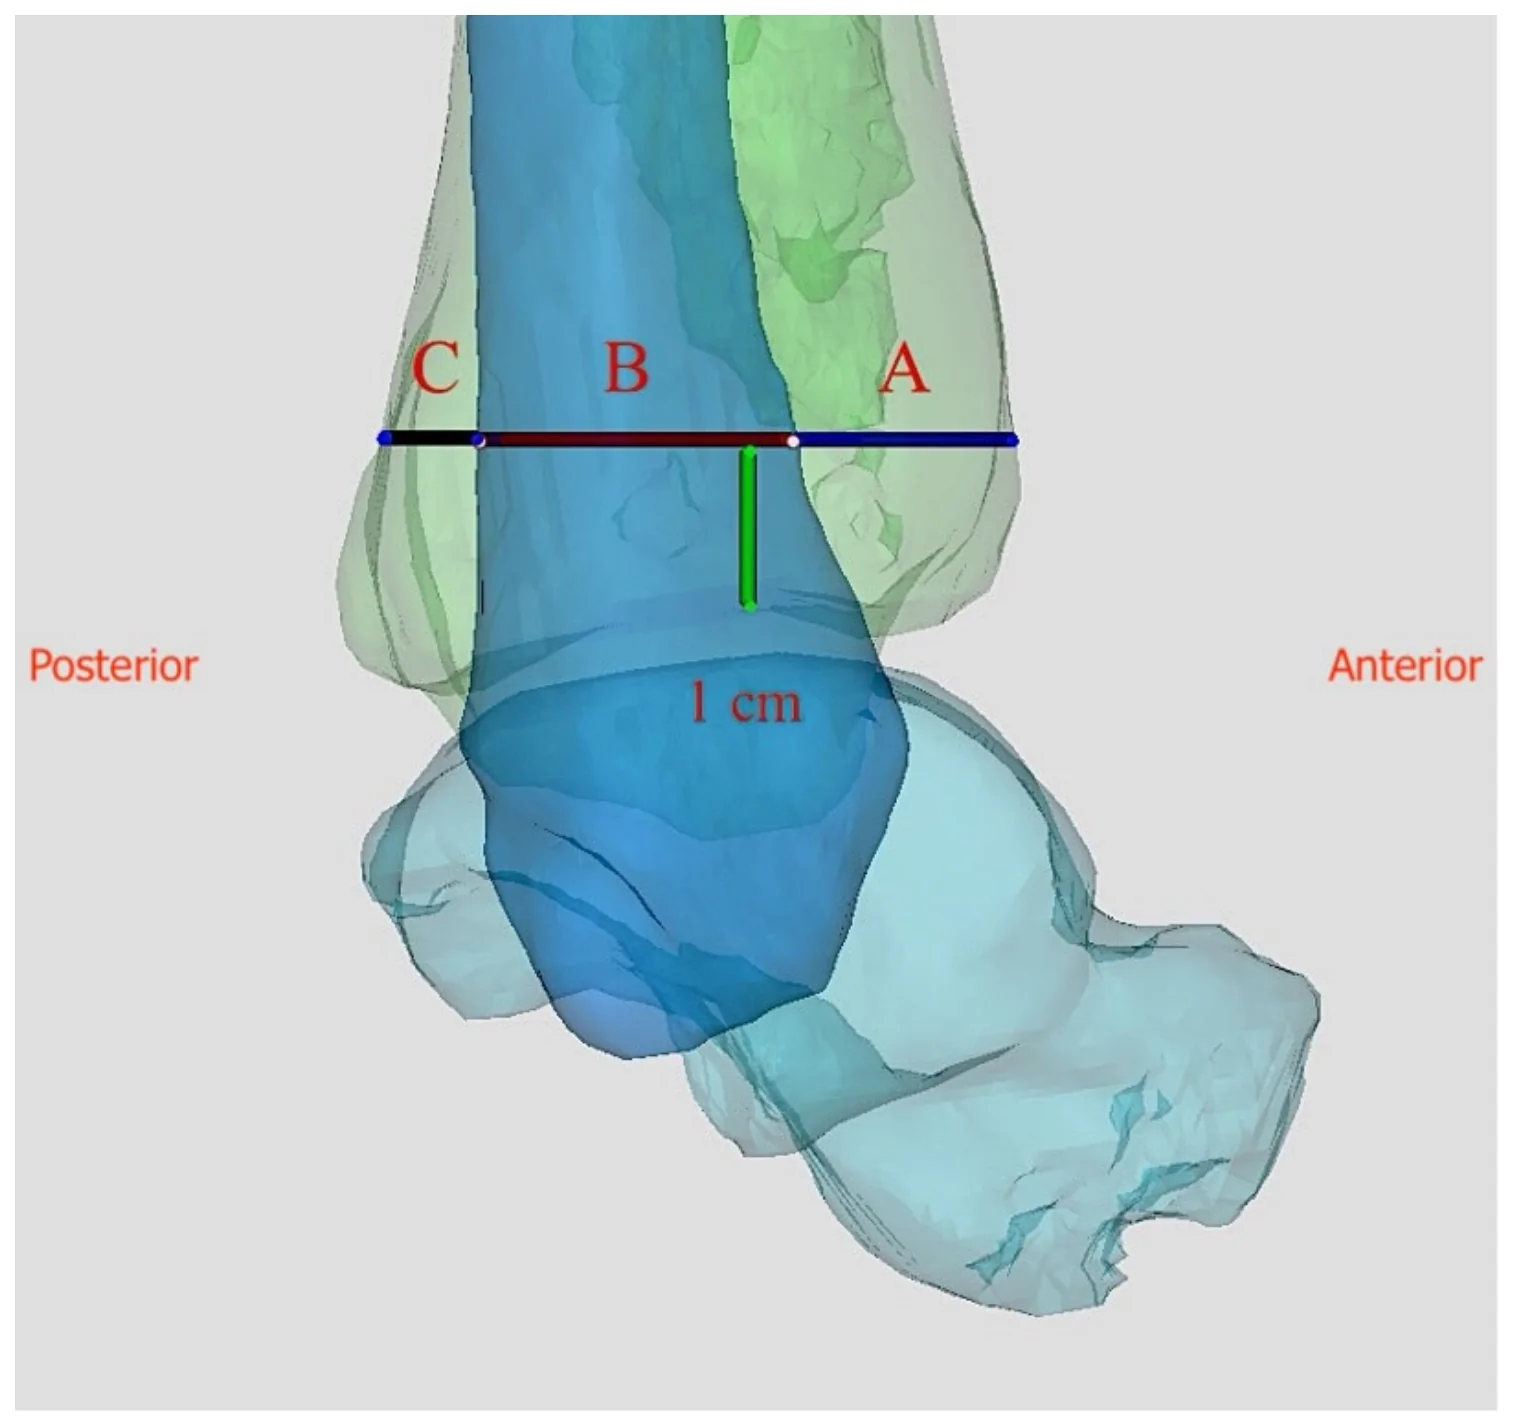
Definition of the lateral measurements in a standardized 3D CT-derived true-lateral projection obtained 10 mm proximal to the tibial plafond. A, distance between the anterior tibial cortex and anterior fibular cortex; B, fibular anteroposterior width; C, distance between the posterior fibular cortex and posterior tibial cortex.
